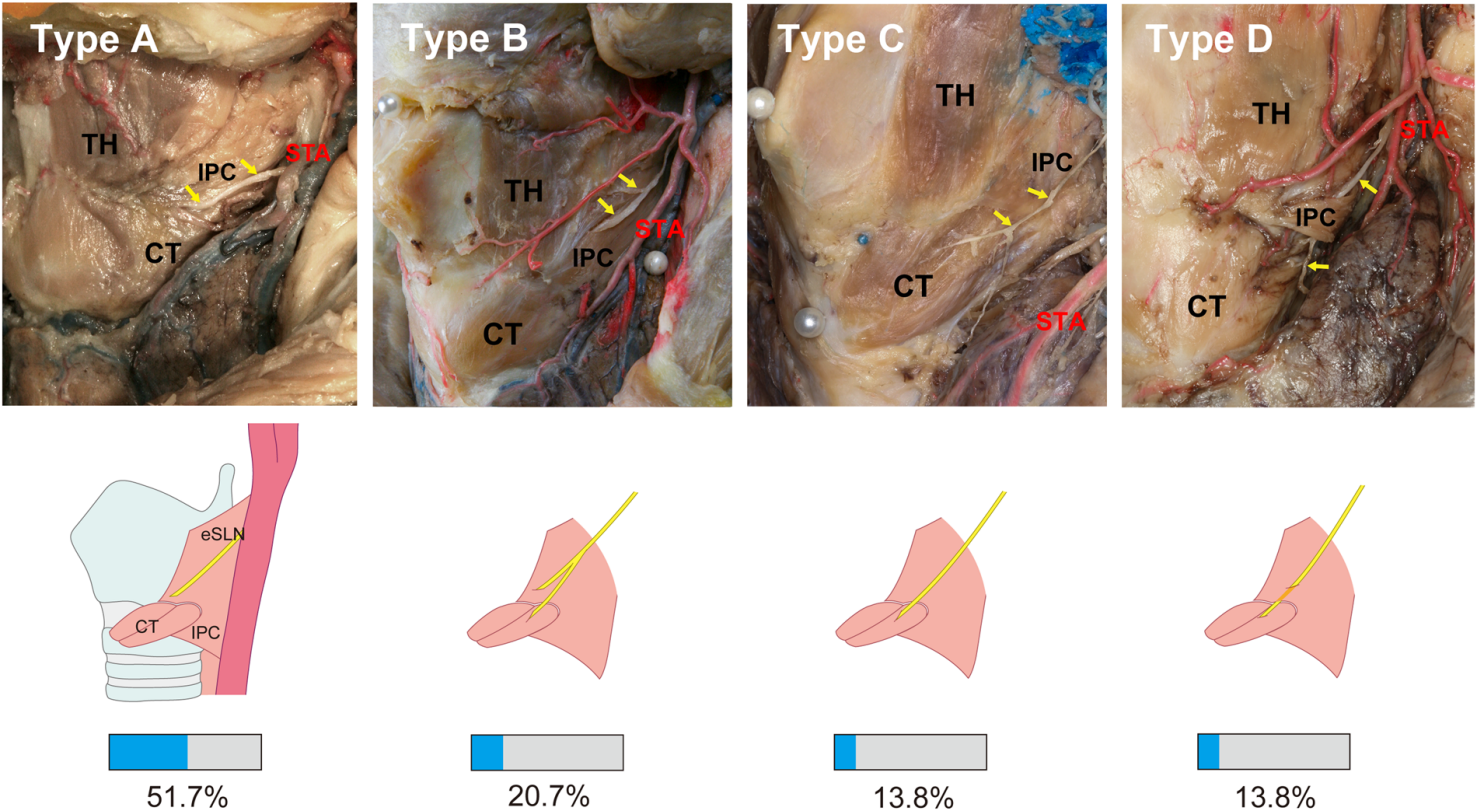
Four patterns of innervation for the external branch of the superior laryngeal nerve (eSLN). IPC, inferior pharyngeal constrictor; CT, cricothyroid; TH, thyrohyoid muscle; STA, superior thyroid artery.
